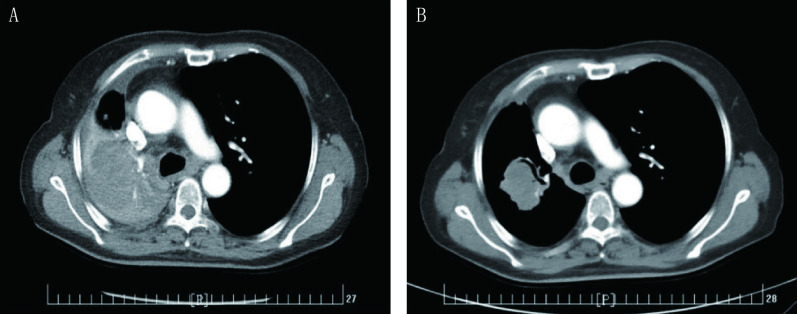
第三次培美曲塞应用。A：第三次应用前基线检查（2017年10月9日）；B：第三次应用最佳疗效（2018年1月2日） The third pemetrexed challenge. A: The baseline CT image before the third pemetrexed challenge (Oct. 9, 2017); B: The best response CT image after the third pemetrexed challenge (Jan. 2, 2018)
